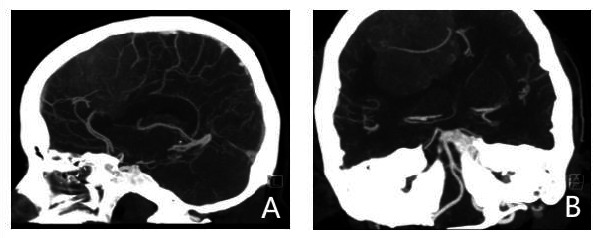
Three-dimensional imaging of intracranial arteries using CT: (A) Sagittal view; (B) Coronal view.
